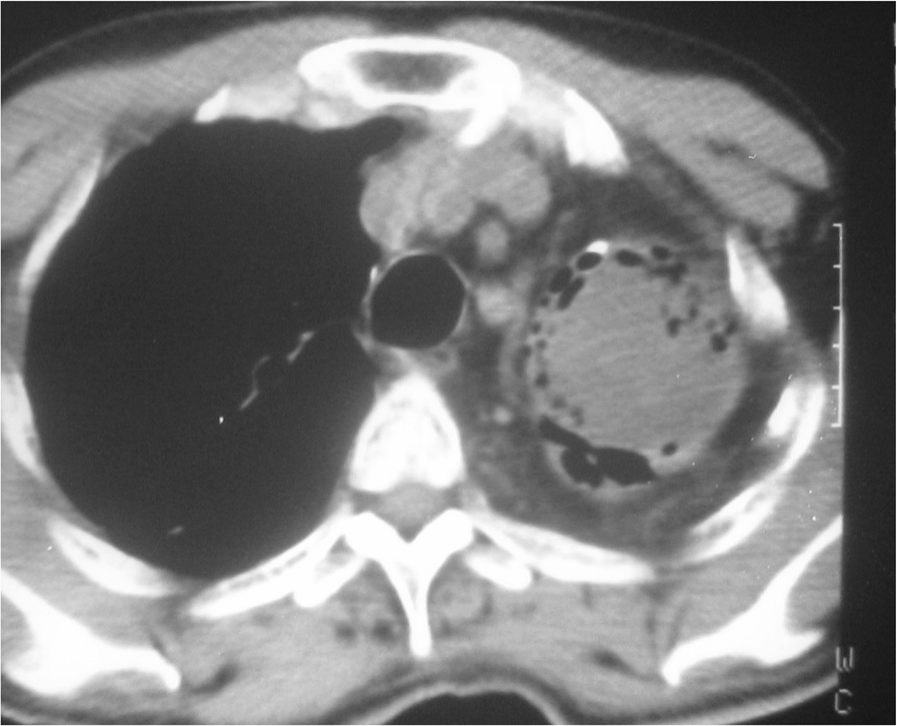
Computed tomographic view of a complex aspergilloma of the left lung
